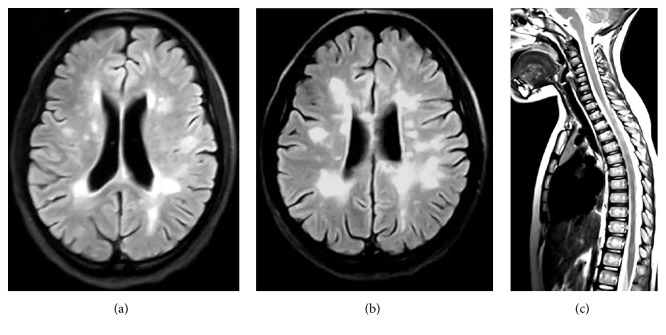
Axial FLAIR weighted images ((a) and (b)) showing multiple hyperintense lesions on periventricular and subcortical regions in patient 5 with spinal dorsal involvement on sagittal T2 weighted image (c).
